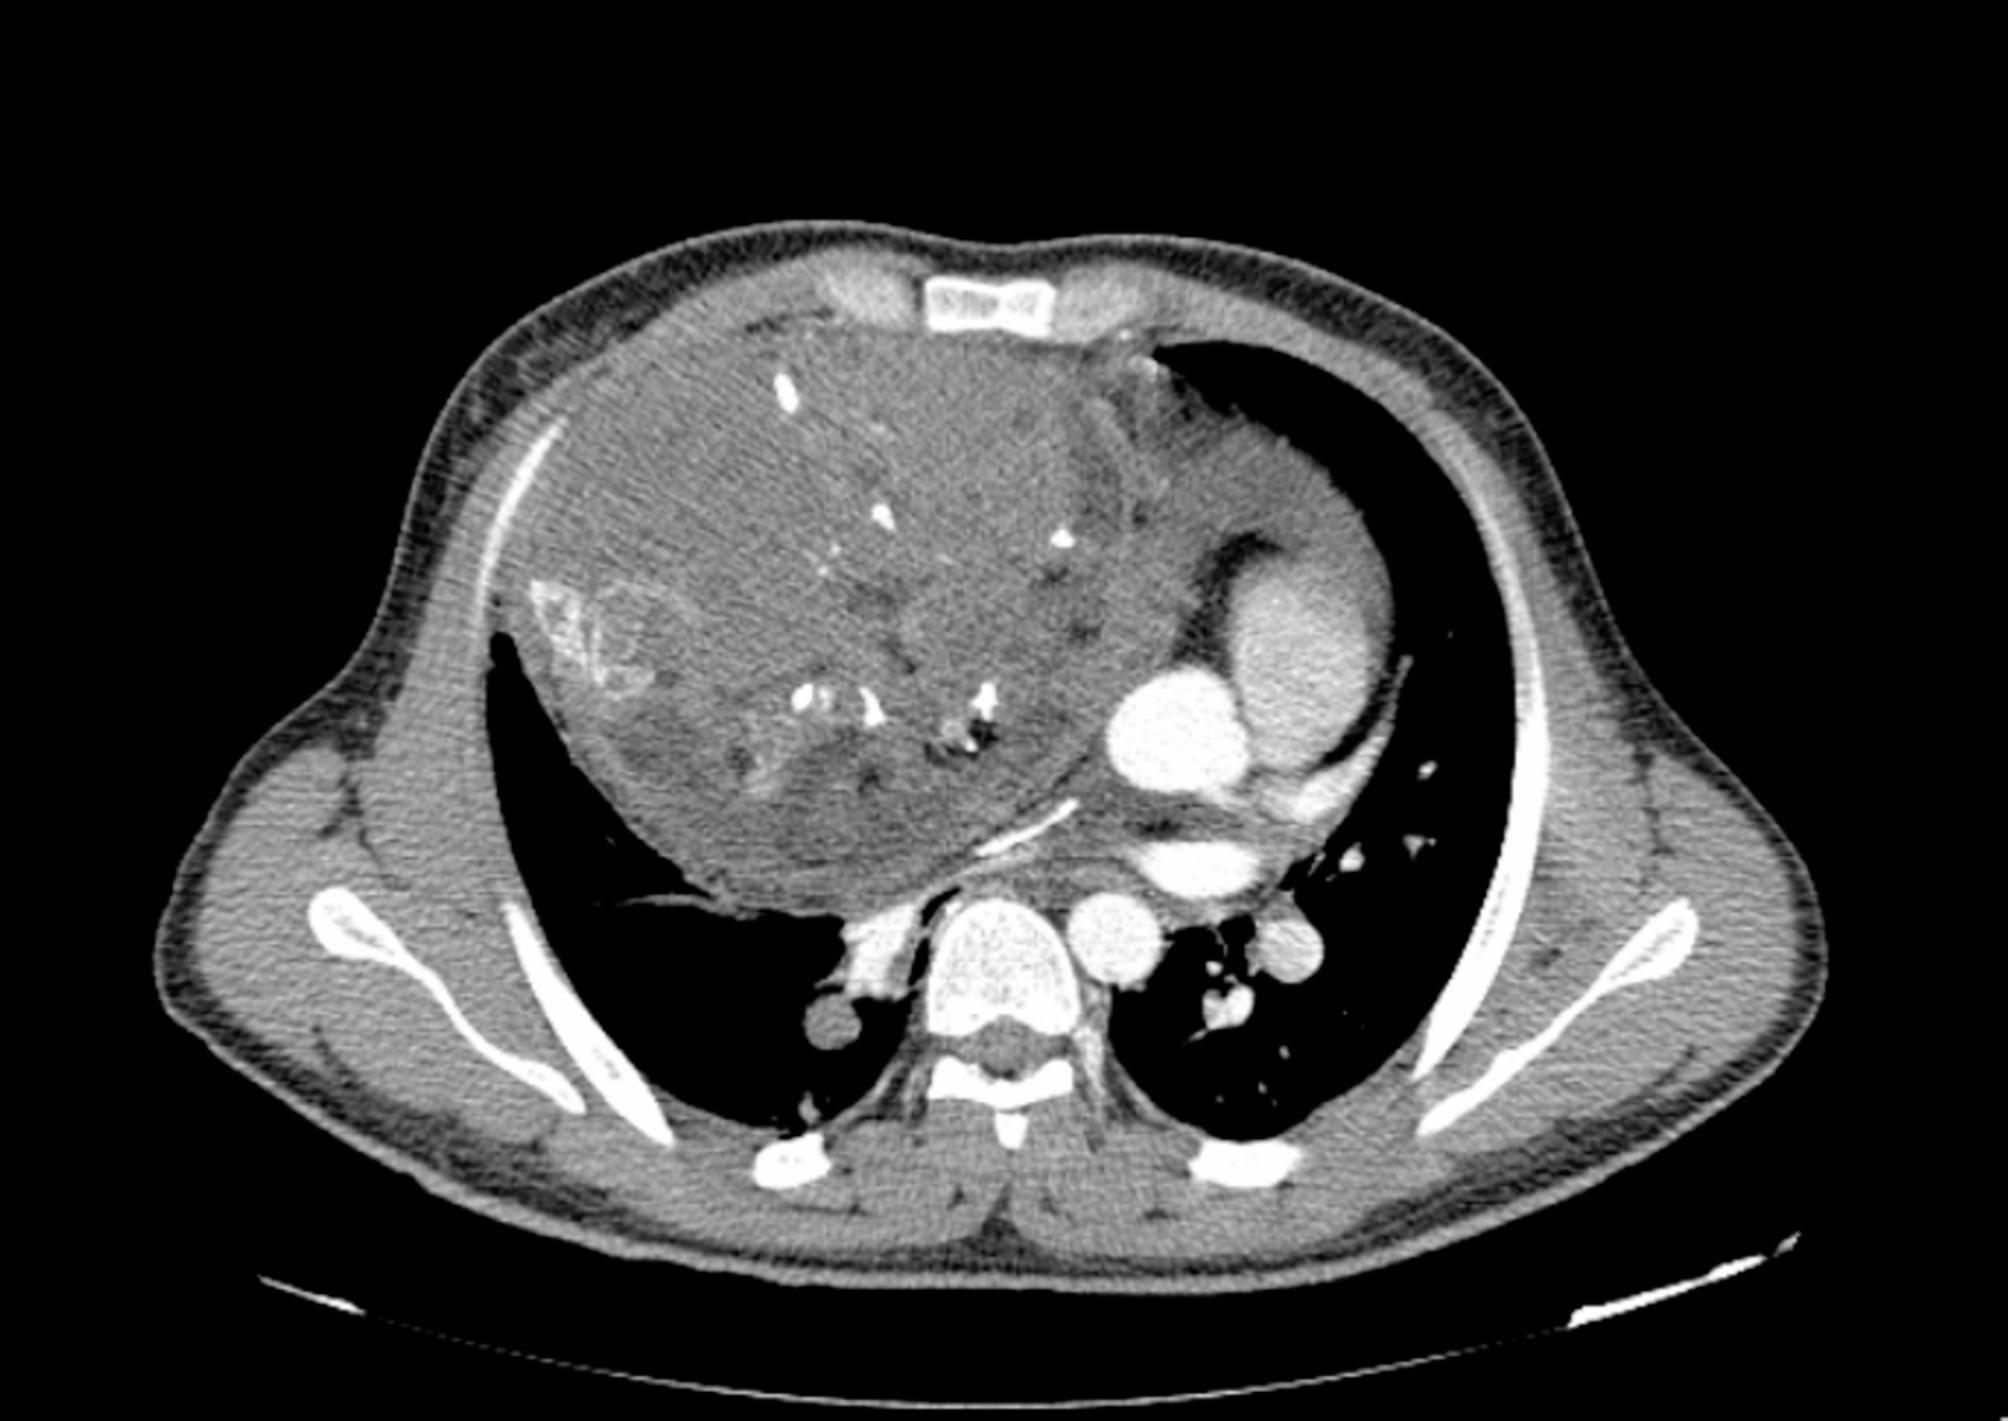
Computed tomography scan showing a large tumor in the anterior mediastinum
